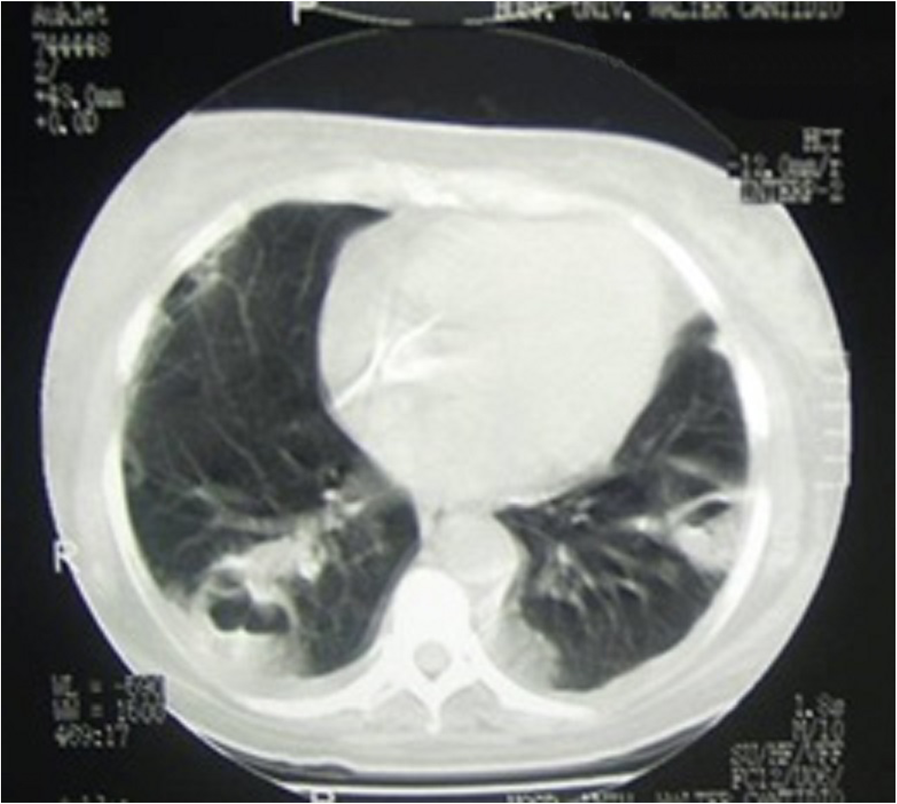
Computed tomography of the thorax with pulmonary lesions with air-fluid levels.
